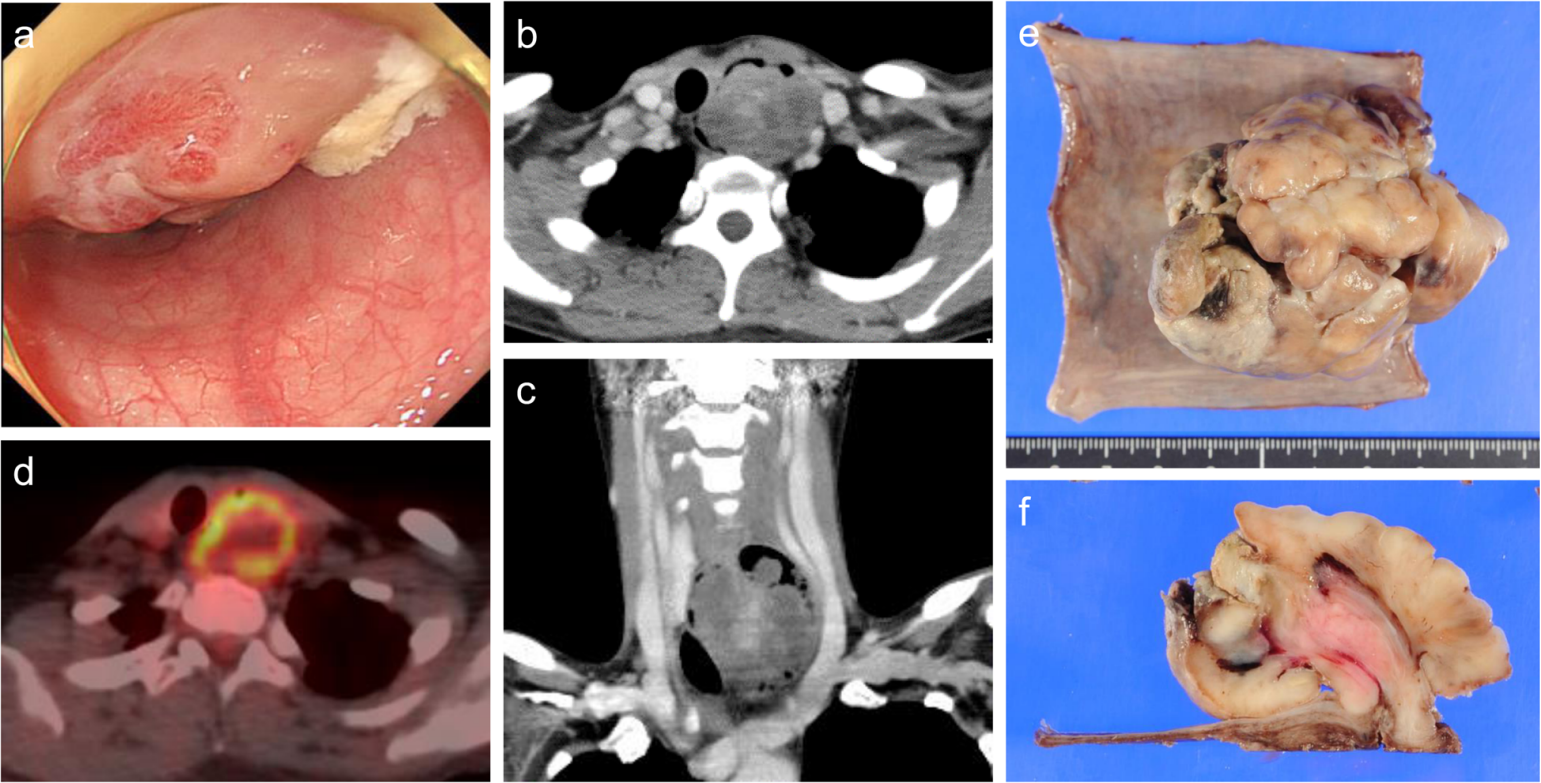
Images obtained before the second surgery. Esophagoscopy showed a 70-mm-sized protruding tumor (a). Contrast-enhanced CT showed a well-circumscribed mass in the cervical esophagus (b, c). FDG-PET/CT showed FDG uptake in the esophageal tumor (d). Macroscopic findings of the secondary tumor. It was pedunculated and multilobulated and covered by the thinning esophageal mucosa (e), while its incised surface was whitish tan and had areas of focal hemorrhage (f)
